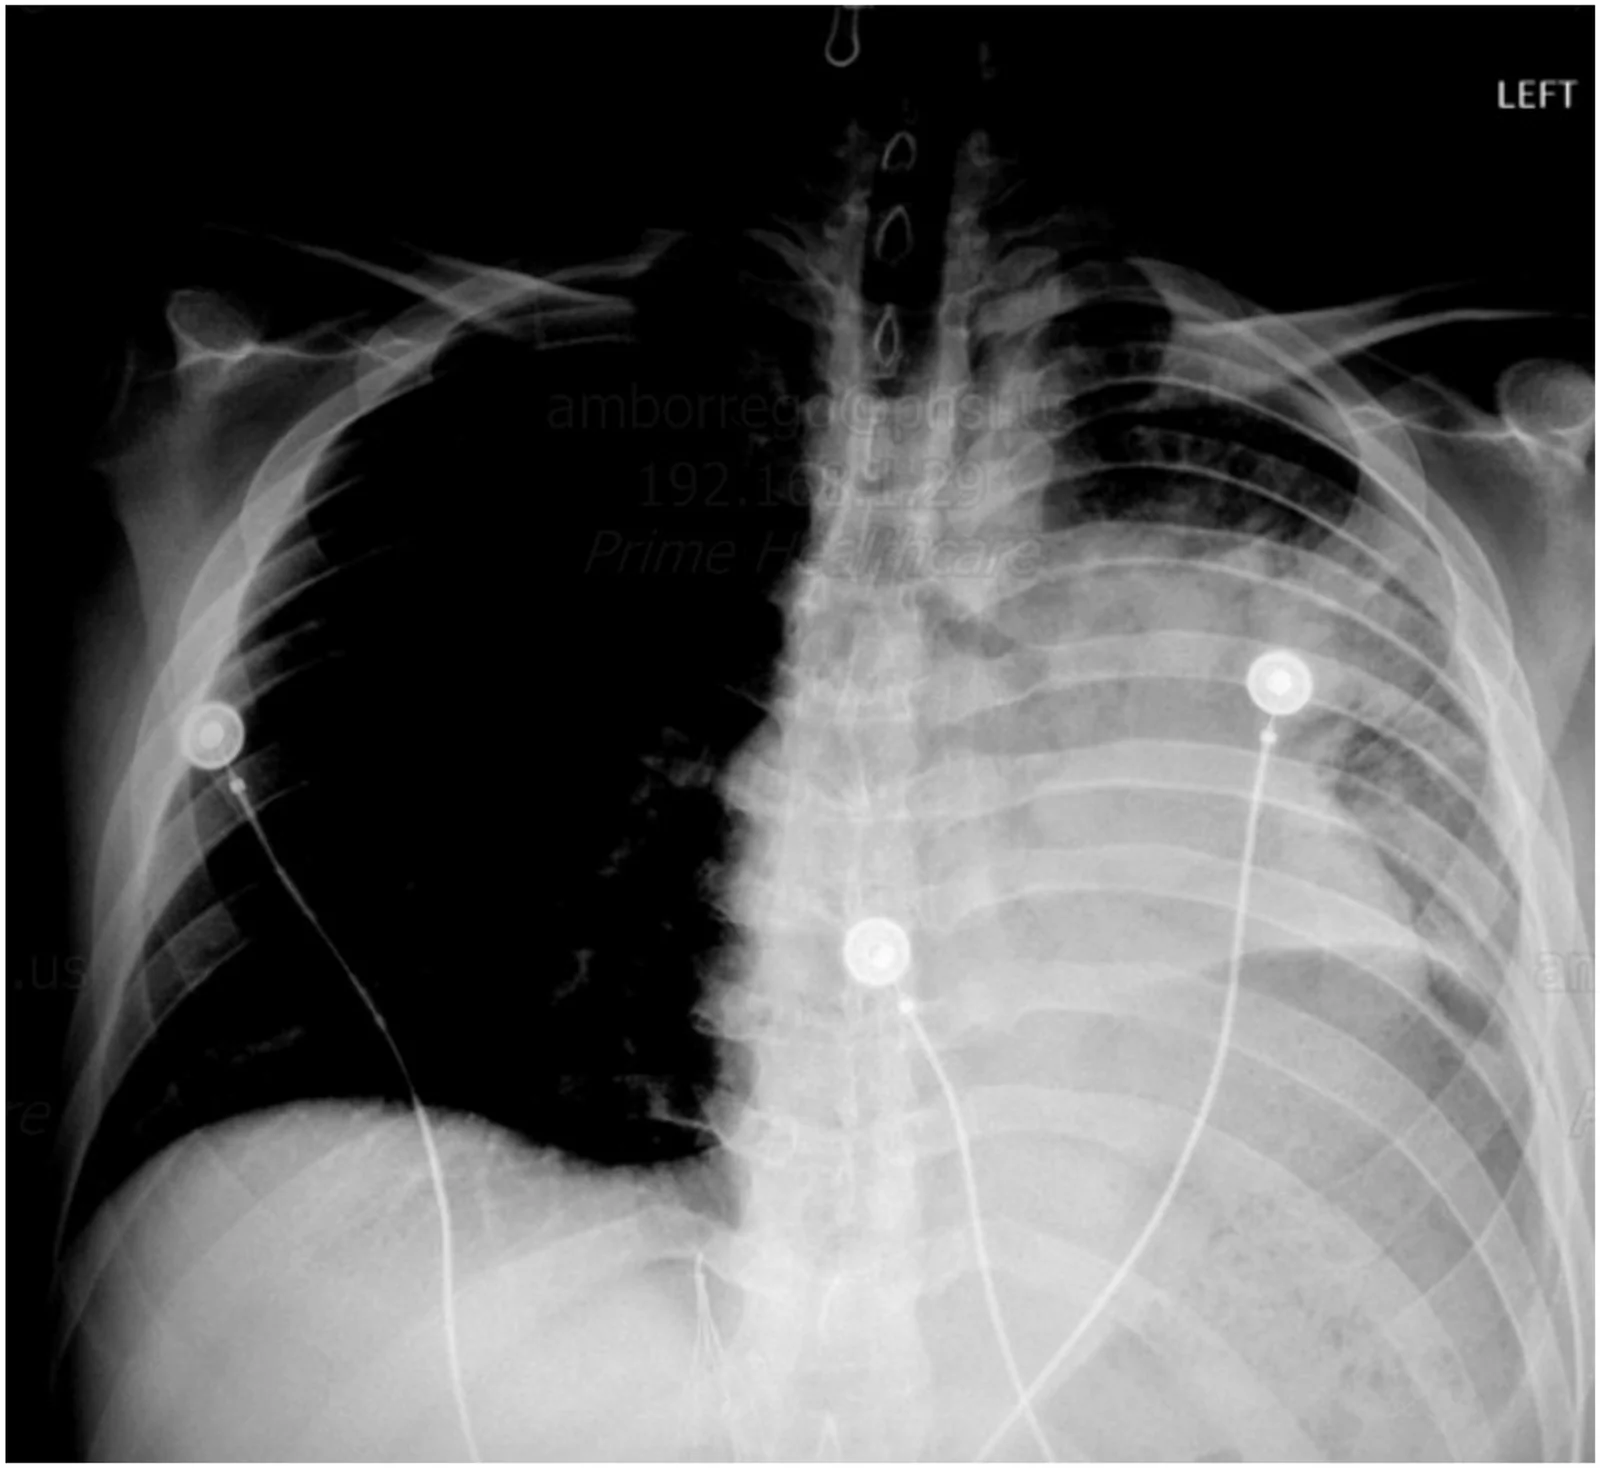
Chest radiograph demonstrating asymmetric left perihilar and lower lobe airspace opacities concerning for an infectious or neoplastic process
